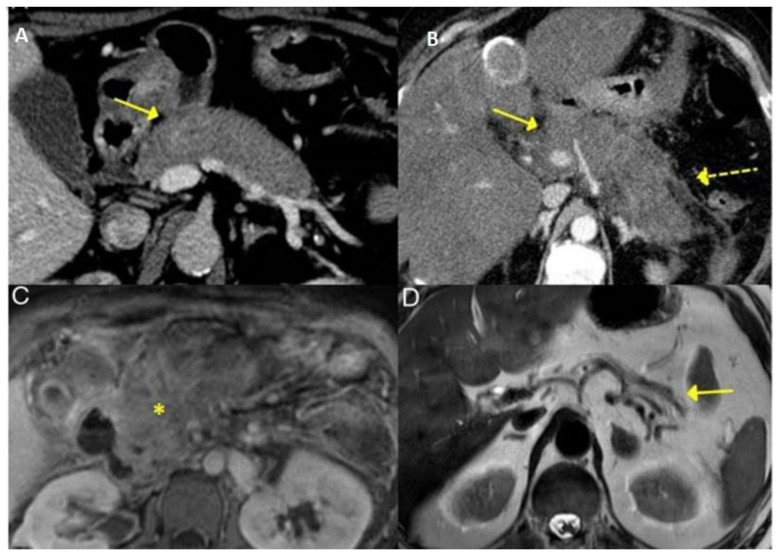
Typical features of AIP 2 in IgG4-RD seen on CT and MRI—Diffuse enlargement of the pancreas at CT (A,B) with blurring of peripancreatic fat (dashed yellow arrow, on B). T1-weighted MRI image of the pancreas (C, asterisk indicates pancreatic fibrosis) showing pancreatic atrophy (solid yellow arrow, D) [Adapted] [23].
